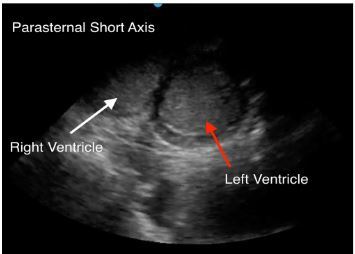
Transthoracic echocardiography: parasternal short view of the heart demonstrating echogenic (thrombosed) blood within the left and right ventricles.
